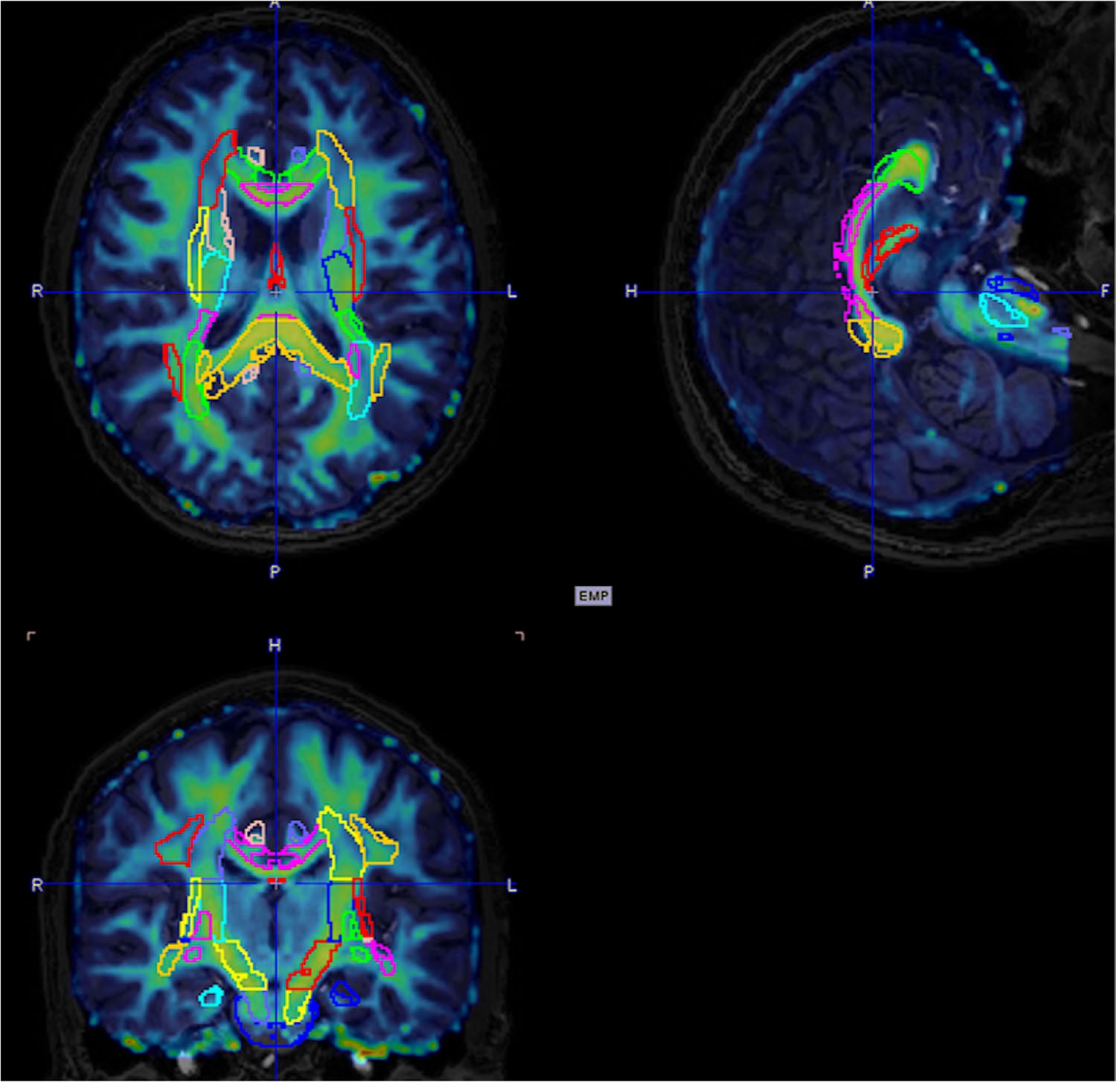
Fused FA/3D T1w MRI image after normalizing to atlas space. The VOIs delineate the white matter regions included in the DTI analyses
